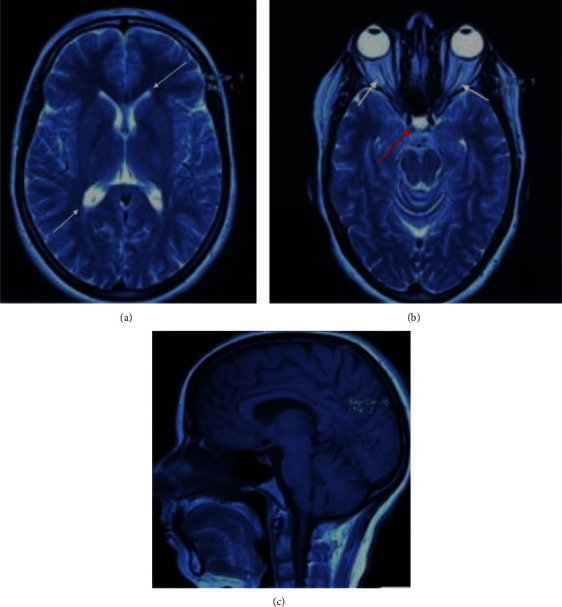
(a) T2 axial sequence MRI showing normal-sized lateral ventricles (white arrow), (b) axial T2 sequence showing optic nerve sheath enlargement (white arrow) with an empty aspect of the sella turcica (red arrow) suggesting intracranial hypertension, and (c) T1 sagittal sequence showing discrete ptosis of the cerebellar tonsils.
